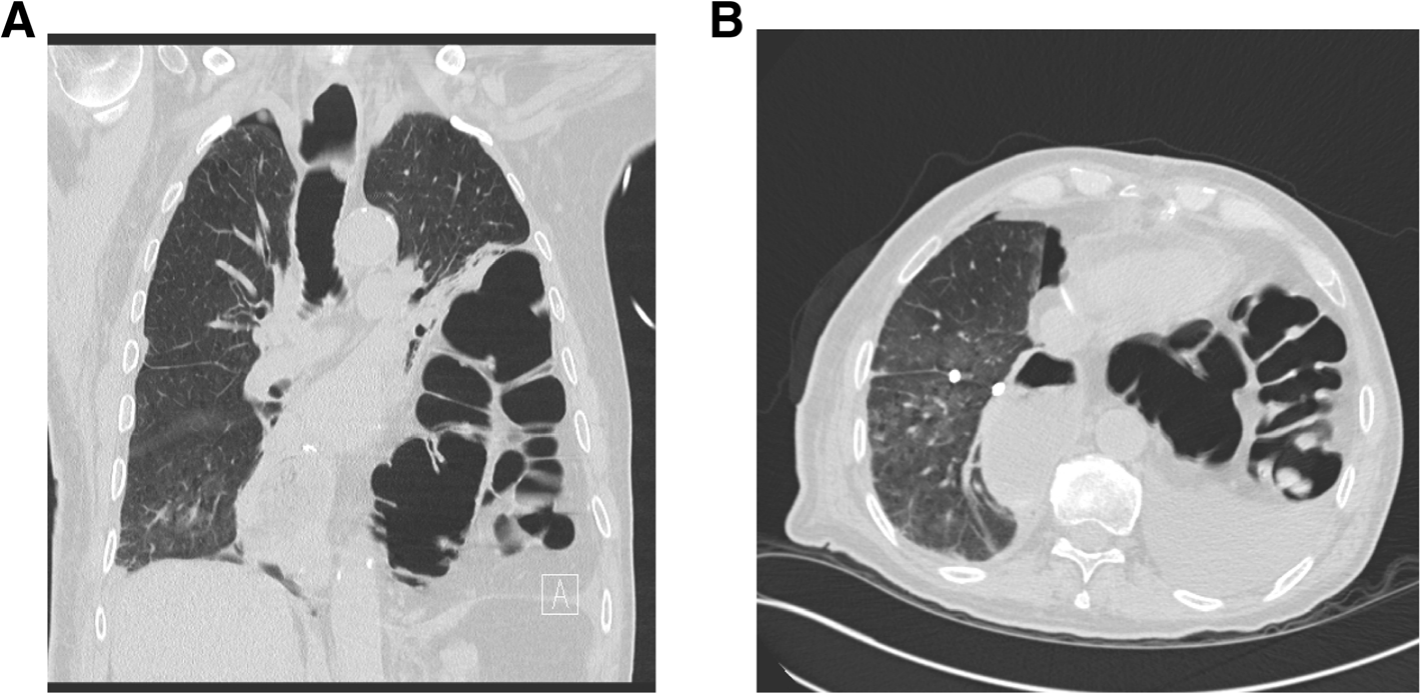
Chest computed tomography depicting left lung atelectasia as a consequence of colic herniation in the chest: a coronal view); (b) Transverse view
